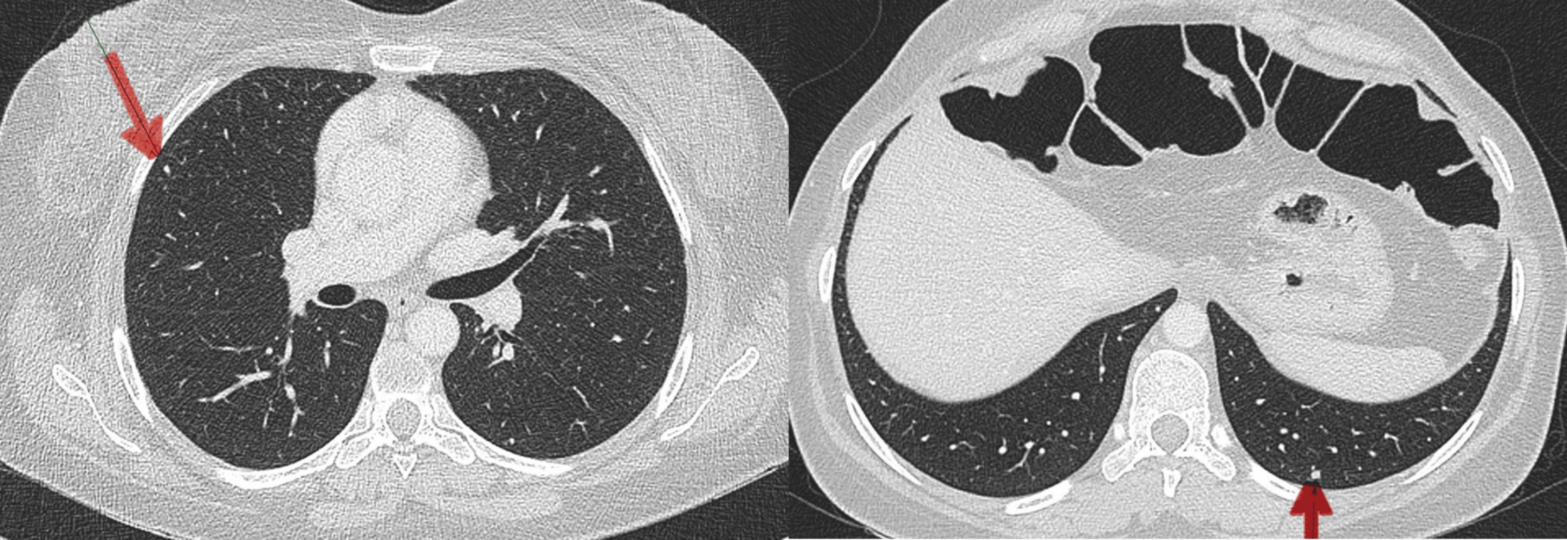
CT scan images. CT scan images showing bilateral small pulmonary nodules. The red arrows indicate small bilateral pulmonary metastases.
